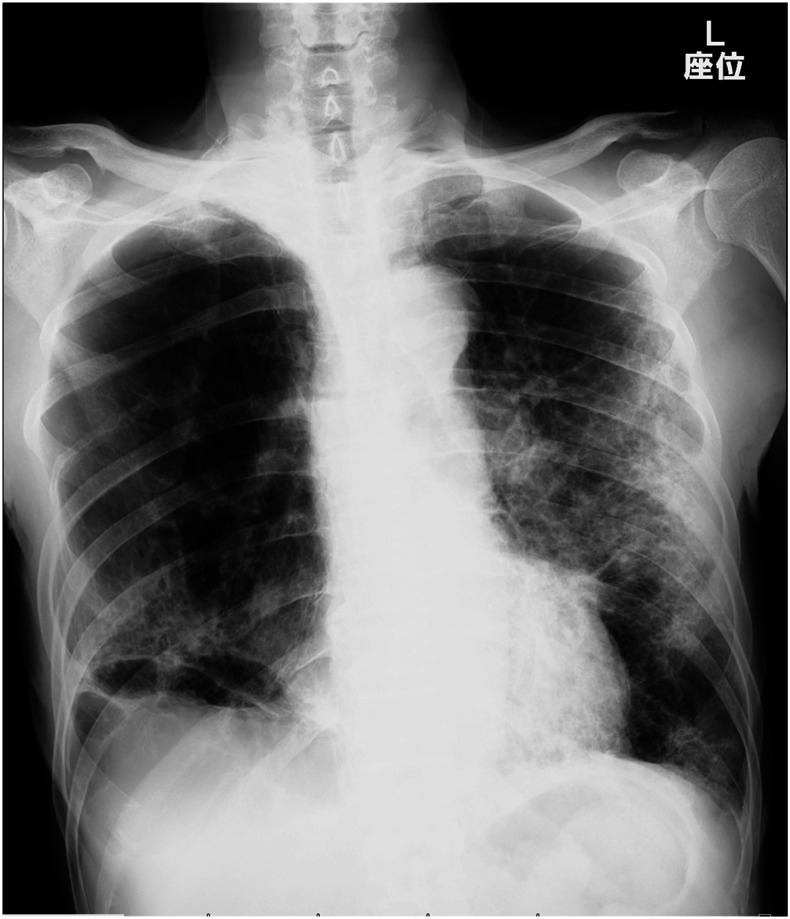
Chest X-ray. A chest X-ray revealed bilateral opacities peripherally.
